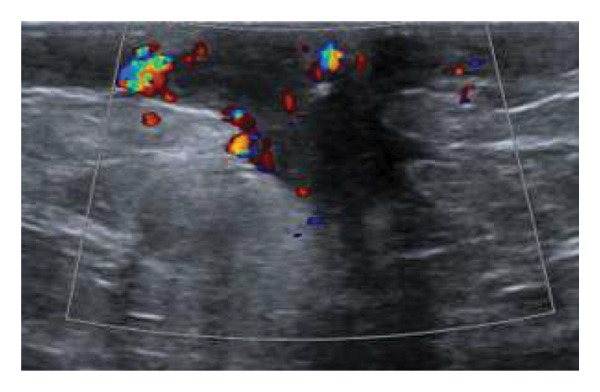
Same patient as in Figure 8. The color Doppler examination shows increased peripheral vascular signals (9).
